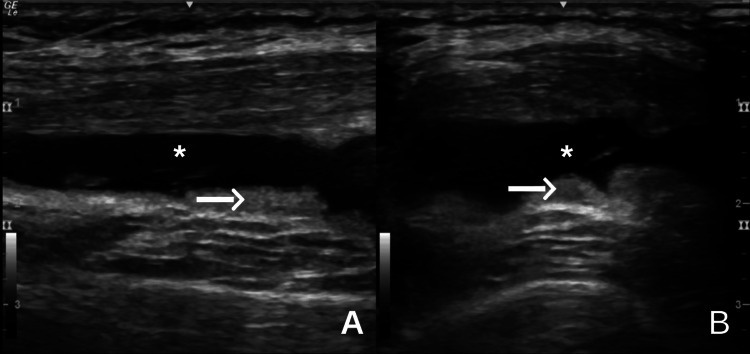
Ultrasound images of the suprapatellar recess in long-axis (A) and short-axis (B) views. The white arrows highlight areas of synovial hypertrophy, while the asterisks indicate the presence of joint effusion, consistent with intra-articular inflammation.
